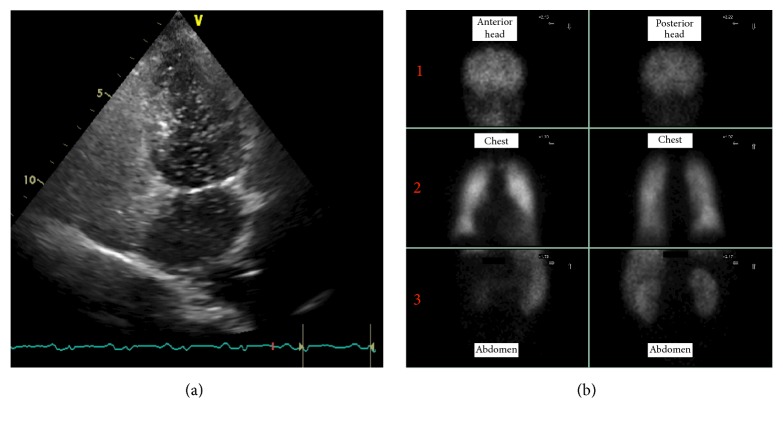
(a) Microbubble transthoracic echocardiography showing a right to left shunt with a delayed opacification (>3 cardiac cycles) of the left atrium and ventricle; (b) macroaggregated albumin lung perfusion scan (MAA scan) showing radioactivity in the lungs (panel 2) as well as in the cerebrum (panel 1) and in the kidneys (panel 3), suggesting right to left shunt.
